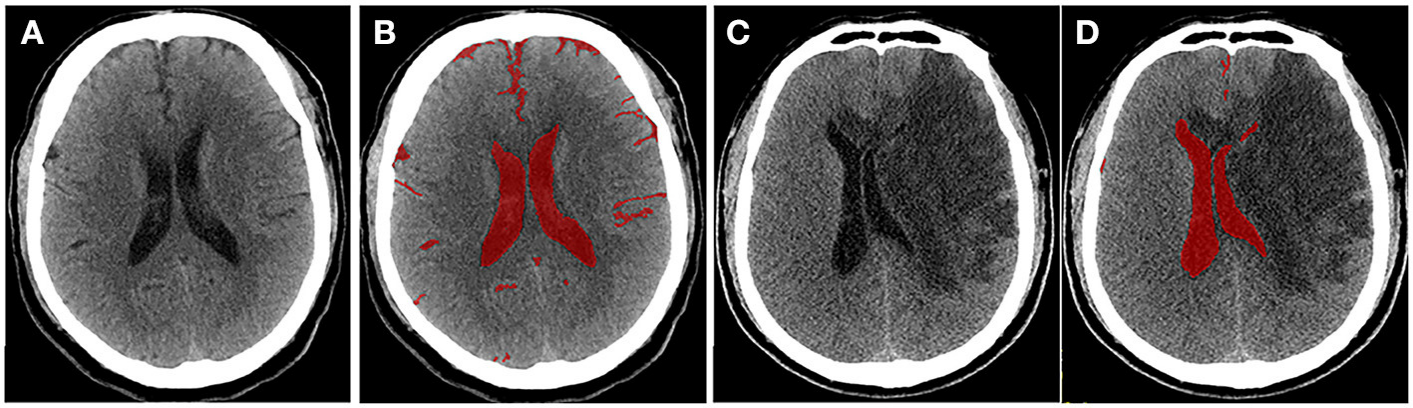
Quantitative cerebrospinal fluid (CSF) shifts. Baseline (A) and 24 h (C) follow-up CTs after ischemic stroke, respectively. CSF spaces are outlined on a CT scan slice at baseline (B) and follow-up (D). Supratentorial CSF and basal cisterns were outlined on each slice, and ΔCSF was subsequently calculated.
